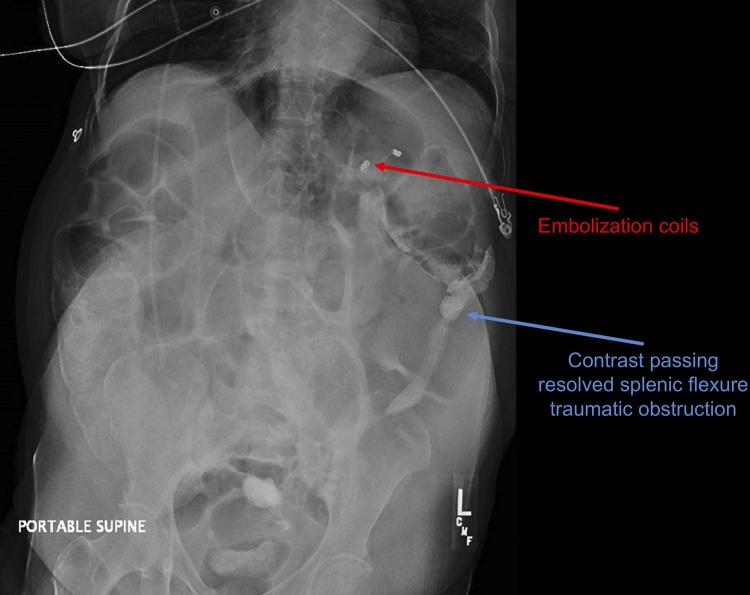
Abdominal x-ray reveals contrast migrating past the area of the splenic hematoma on post-splenic artery embolization day six.
